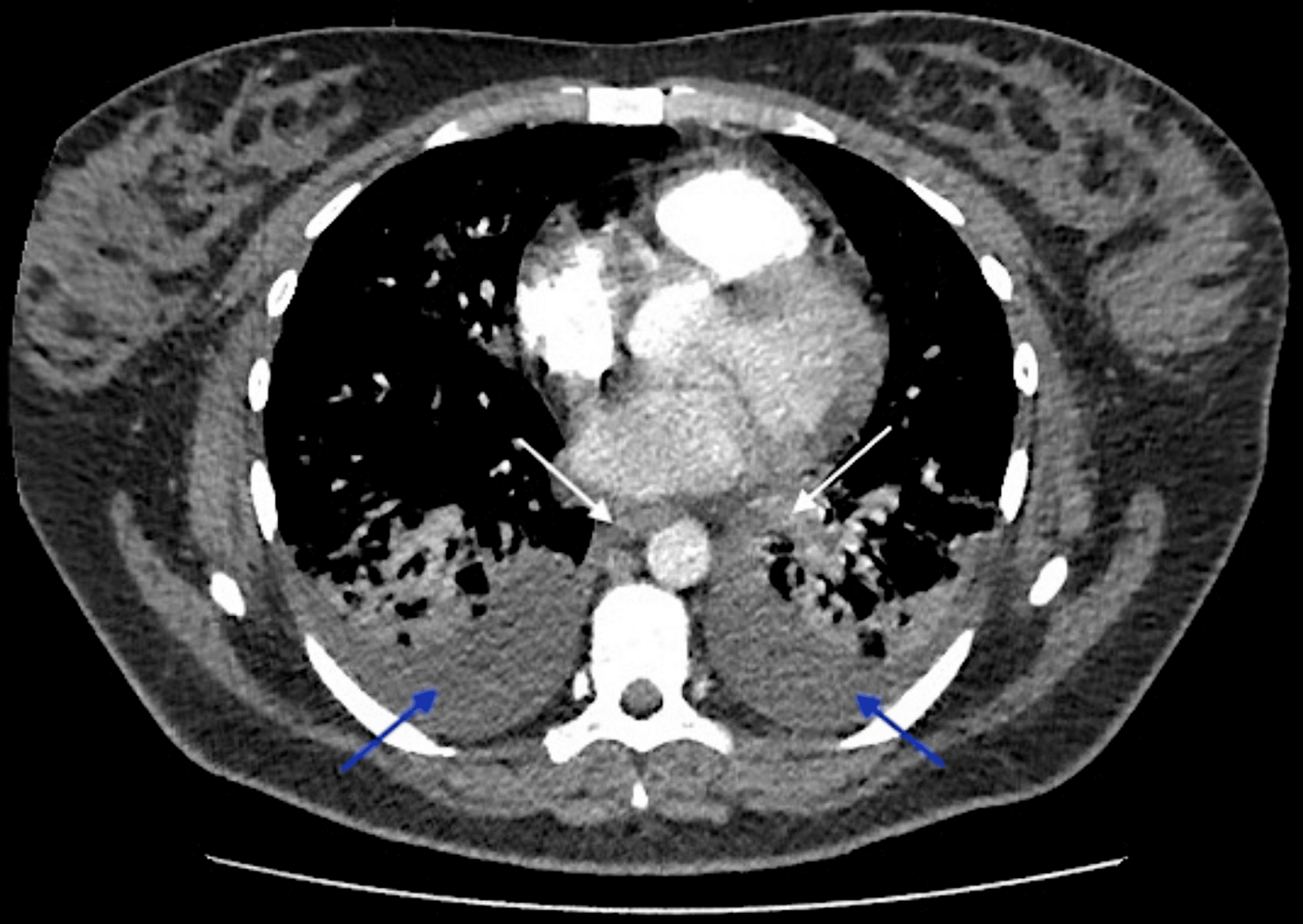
Chest CT scan Bilateral perihilar and centrilobular confluent consolidation along with small bilateral pleural effusions. Importantly, the pulmonary artery and its segmental branches were widely patent, showing no signs of pulmonary embolism or significant pericardial effusion. Additionally, there was no evidence of hilar or mediastinal lymphadenopathy, nor any notable bony abnormalities. These findings were consistent with bilateral bronchopneumonia and reactive effusion, effectively ruling out pulmonary embolism. (Arrows are added to highlight the bilateral perihilar consolidation located in the central part of both lungs near the hilum, annotated with white arrows. Additionally, the small bilateral pleural effusions are indicated at the lower posterior regions of both lungs, annotated with blue arrows.)
